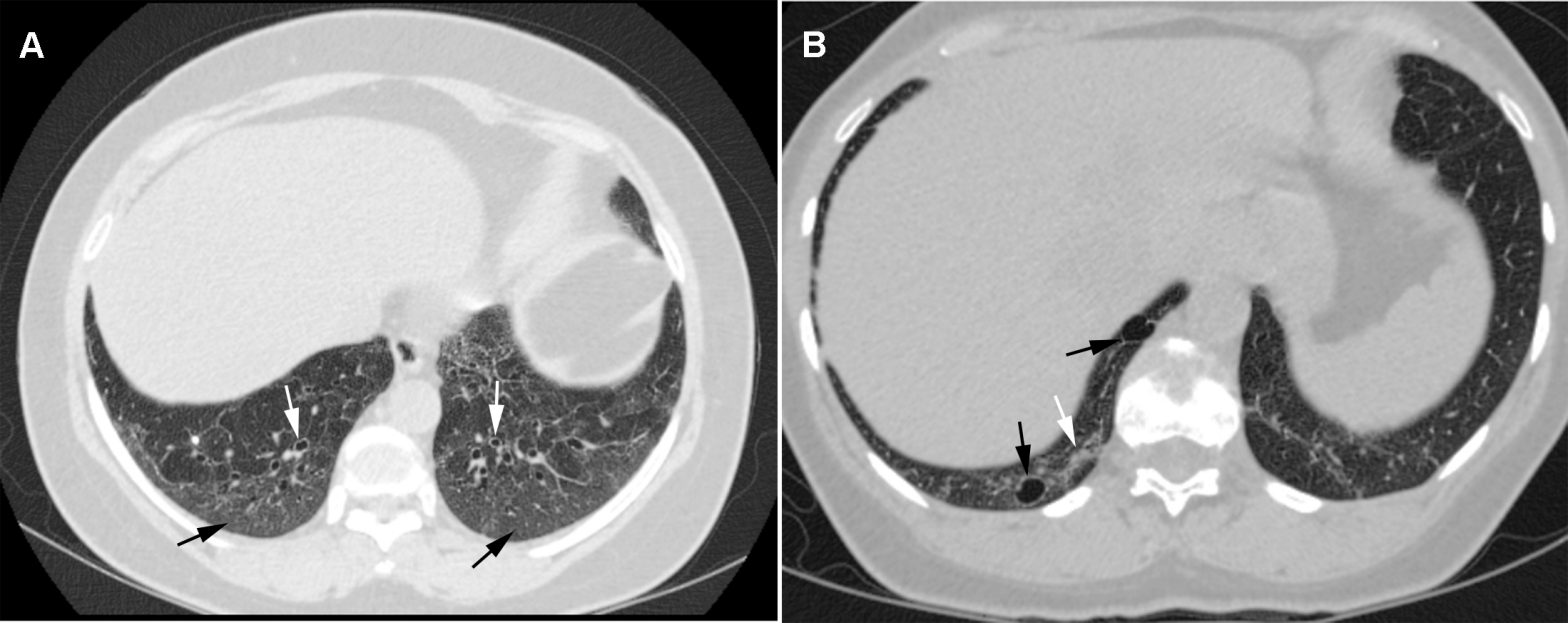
Transverse CT scans obtained from two women aged 60 and 47 years, respectively, suffering from HAM/TSP. a) Bronchiectasis signet ring appearance (white arrows) and ground-glass opacity (black arrows) b) ground-glass opacity (white arrow) and a pulmonary cyst in the right lower lobe (black arrows).
